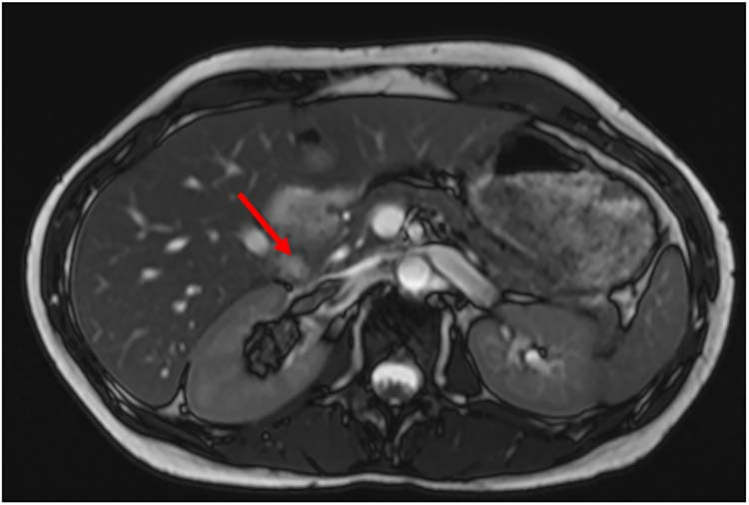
Preoperative MRI with tumor thrombus extension into right renal vein.
